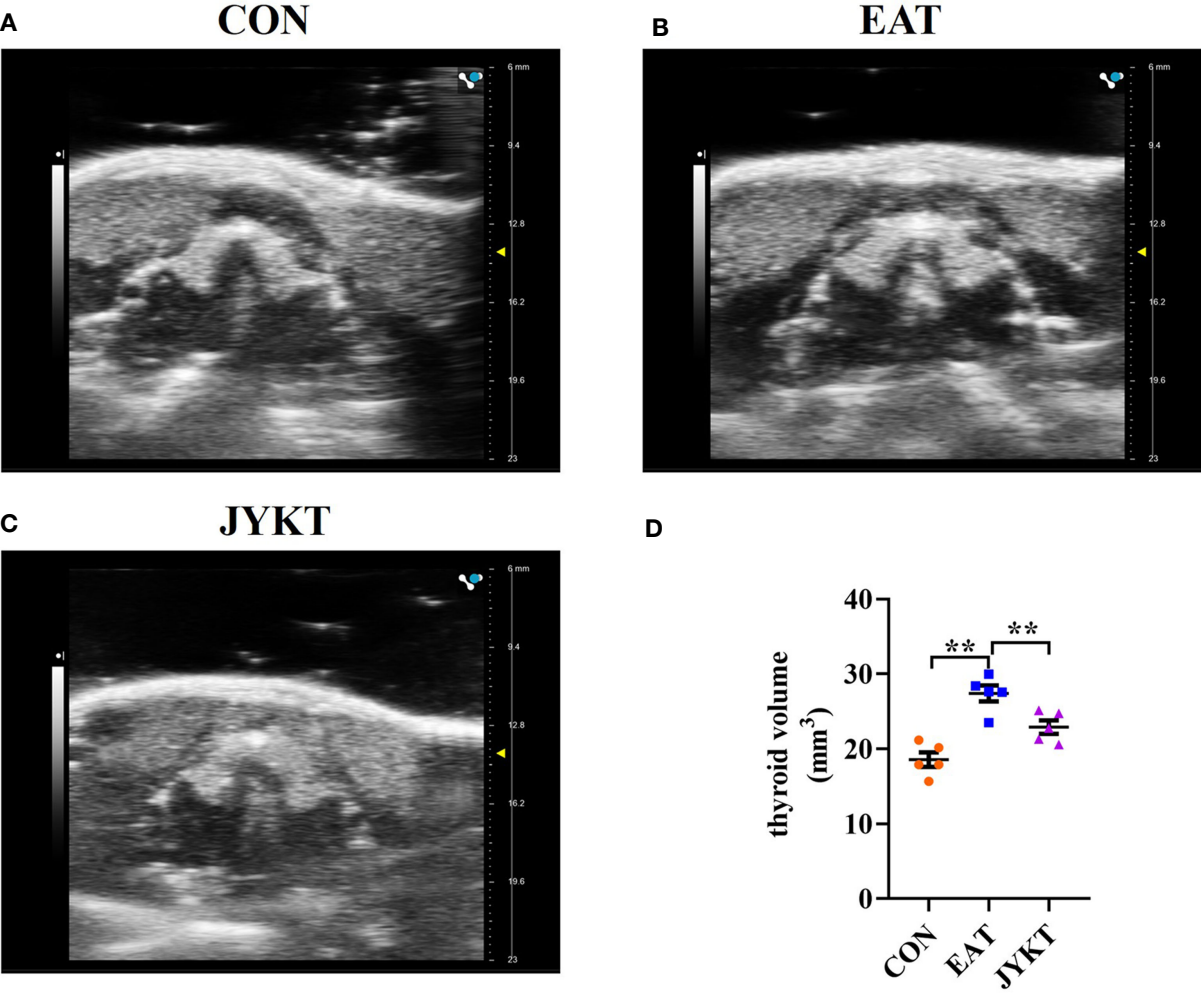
Morphology of thyroid in the CON (A), EAT (B) and JYKT group (C) by ultrasonography. (D) Thyroid volumes among groups. ^^
